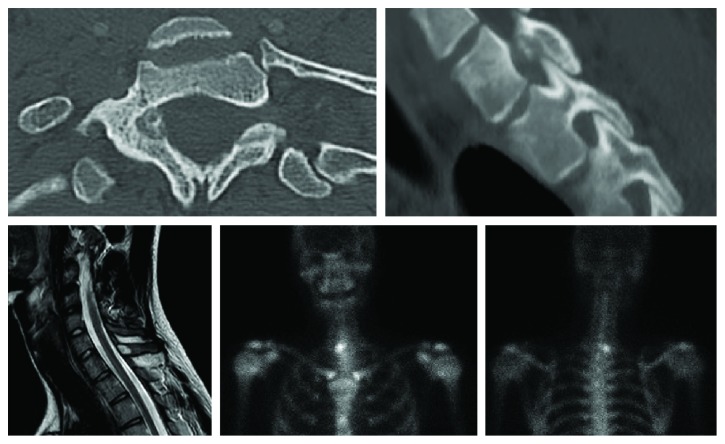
CT scan and MRI of the spine and bone scan. The CT scan shows a rounded osteolytic lesion surrounded by sclerotic bone. Its size is inferior to 15 mm of diameter, within a nidus. MRI shows a T2 hypersignal and a T1 hyposignal on the right side of the T1 vertebra, until the vertebral body with an attachment to the pedicle, and an inflammation of the right T1 root. The bone scan shows hyperfixation of the right T1 vertebra region.
